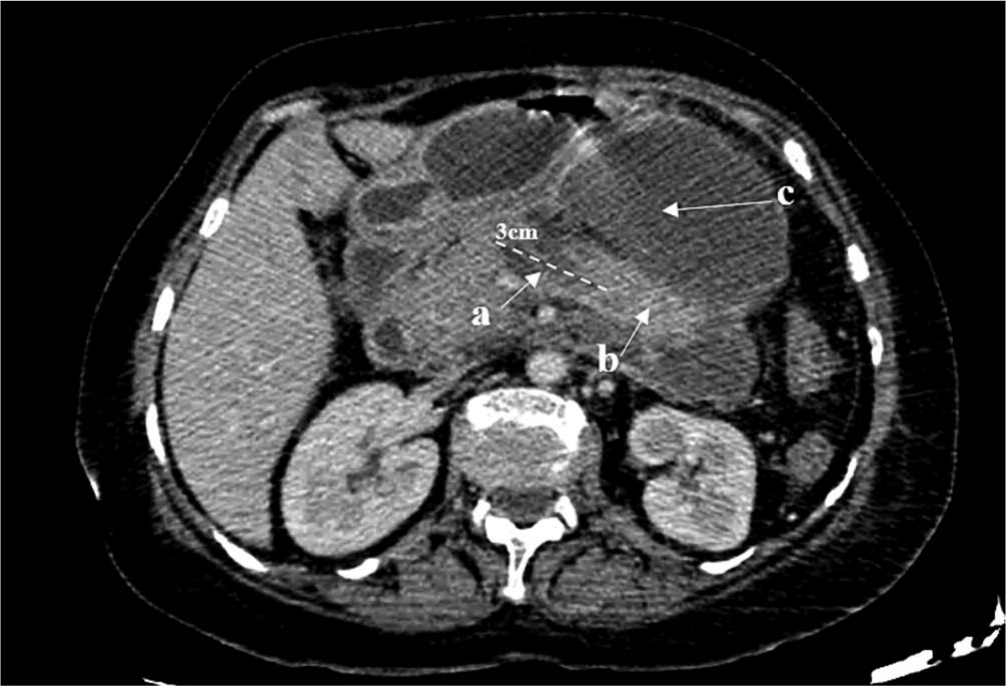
Axial CT scan showing a fluid collection occupying the body (a), measuring 3 cm, with a viable upstream segment (b). Multiple peripancreatic walled-off necrosis (c).
